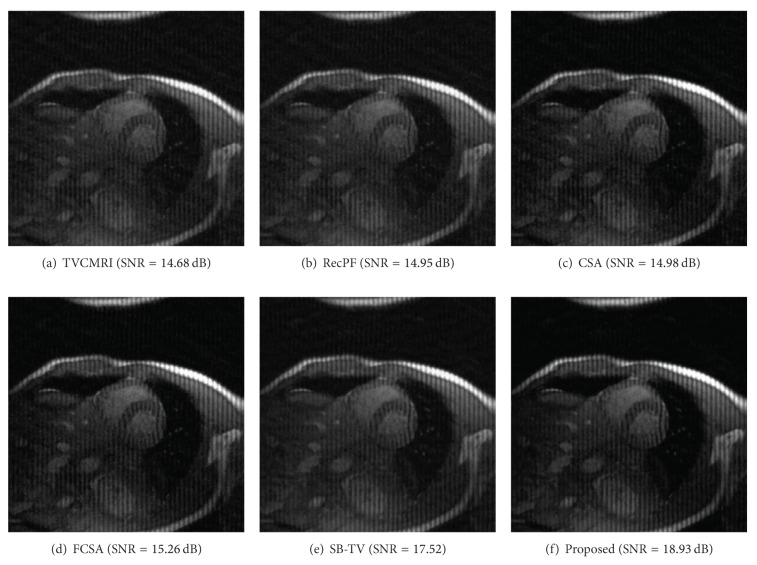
Cardiac MR image reconstruction from 20% samples (Rician noise case).
